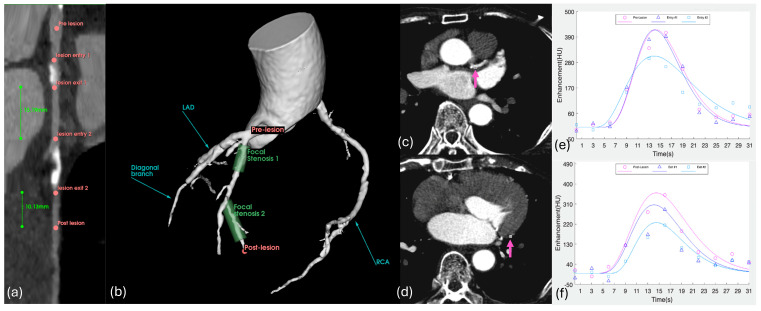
An 80-year-old male (patient 14 in Table 1) whose left circumflex artery (LCX) had two 75% focal stenoses apart from each other by 1.5 cm. The FFR value measured with CT- DAI below the second stenosis in the distal LCX segment (coronary segment #13) was 0.87. In comparison, the FFR value acquired with the invasive method in the same coronary segment was 0.90. (a) Straightened lumen view of the LCX with the sampling ROIs. Two pairs of lesion entry and exit ROIs were placed between the pre-lesion and post-lesion ROIs. (b) Locations of the stenoses and sampling ROIs shown in the three-dimensional image of the LCX. (c) Pre-lesion ROI (pink arrow) and (d) post-lesion ROI (pink arrow) shown in the axial CT myocardial perfusion image at one time point. (e,f) The plots reveal the raw data points sampled from the ROIs shown in (a–d) and the corresponding fitted coronary time-enhancement curves.
